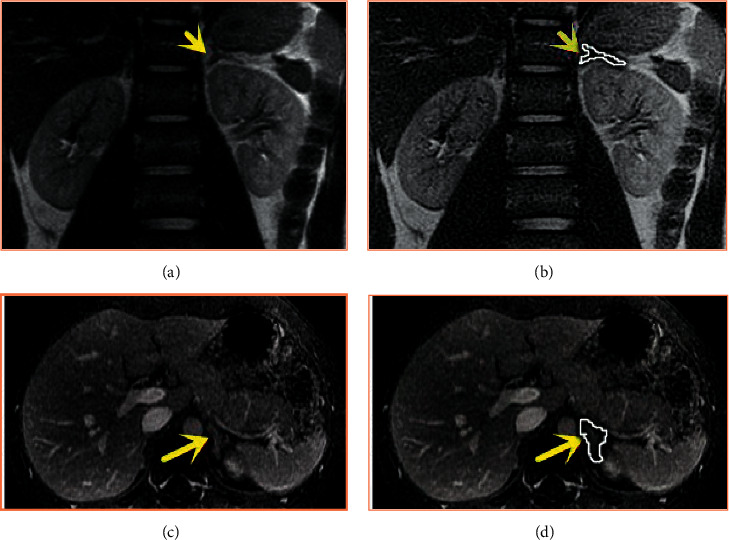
Image processing. (a, b) MRI images of different sequences before processing. (c, d) MRI images processed by BPSO algorithm. The yellow arrow refers to the location of the tumor.
